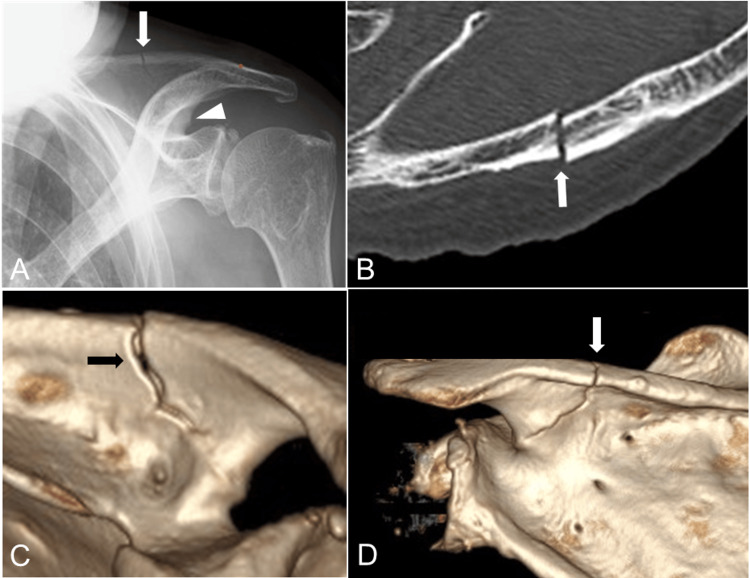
Radiological findings at the patient's initial visit Plain radiography (A) and CT (B, axial view; C, anteroposterior view of 3D-CT; D, posteroanterior view of 3D-CT) shows a transverse fracture (arrows) of the scapular spine, but the alignment is almost normal, and a beak was formed at the scapular notch (arrowhead).
